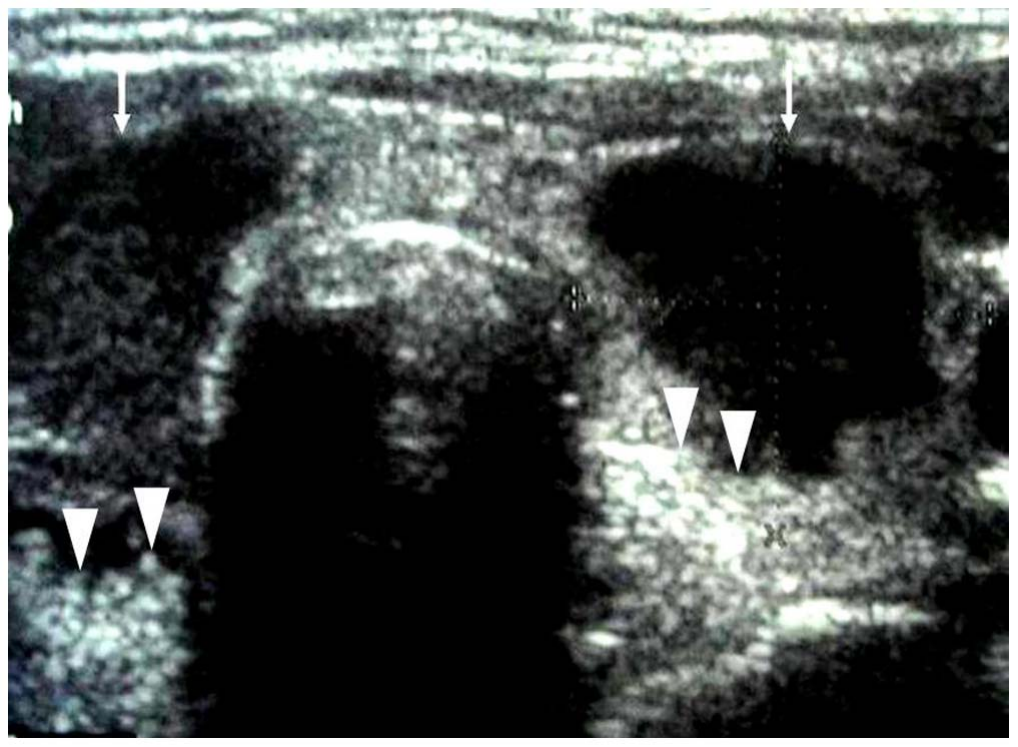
A 78-year-old female patient with non-diffuse PTL. The transverse sonogram shows the extremely hypoechoic lesions (arrows) with posterior acoustic enhancement (arrow heads).
